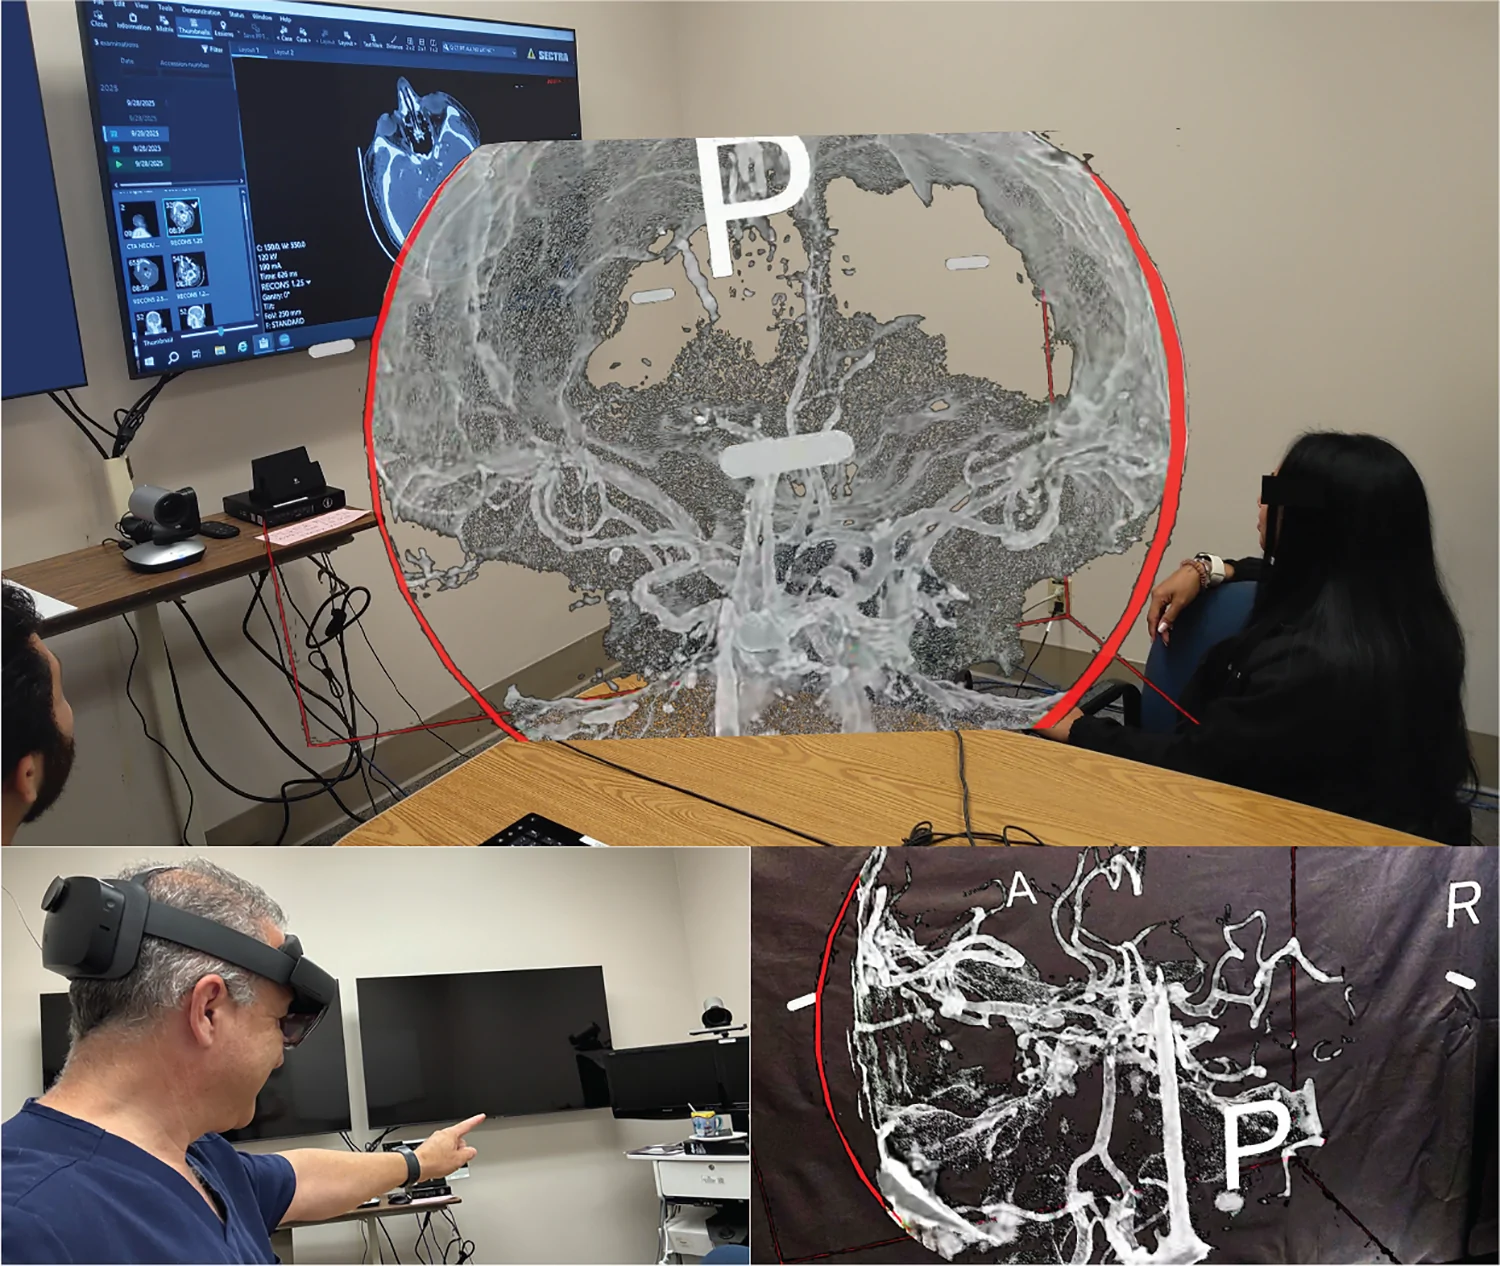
Holo-PACS set-up [Holo-PACS directory and CTA hologram views]. Figure 1 shows the Holo-PACS setup. (Top) CTA Hologram as viewed during weekly clinical case conferences, in stroke team conference room. This imaging is used for educational purposes to view 3D structure and LVOs, not for clinical diagnosis. (Bottom Left) View of provider wearing the HoloLens2 Head-Mounted-Display (HMD) and interacting with the Holo-Stroke-CTA hologram in the conference room. (Bottom Right) Holo-PACS Holo-Stroke CTA Hologram as rendered through the HoloLens2 viewer.
